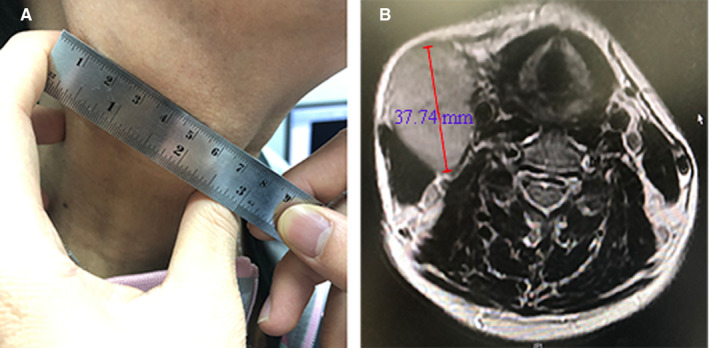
An example of different measurement methods and results of the same lymph node. A patient aged between 40 and 60 y old was diagnosed as N3 NPC, with the greatest diameter of the cervical lymph node = 6.50 cm by palpation (A); while in MRI, the maximal axial diameter of the same node = 37.74 mm (B)
